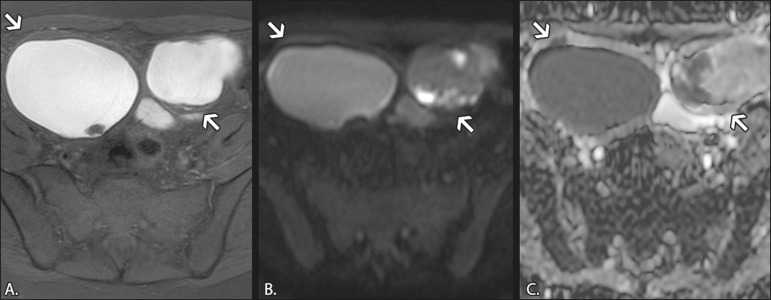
MRI of a 41-year-old female patient with endometriomas.
